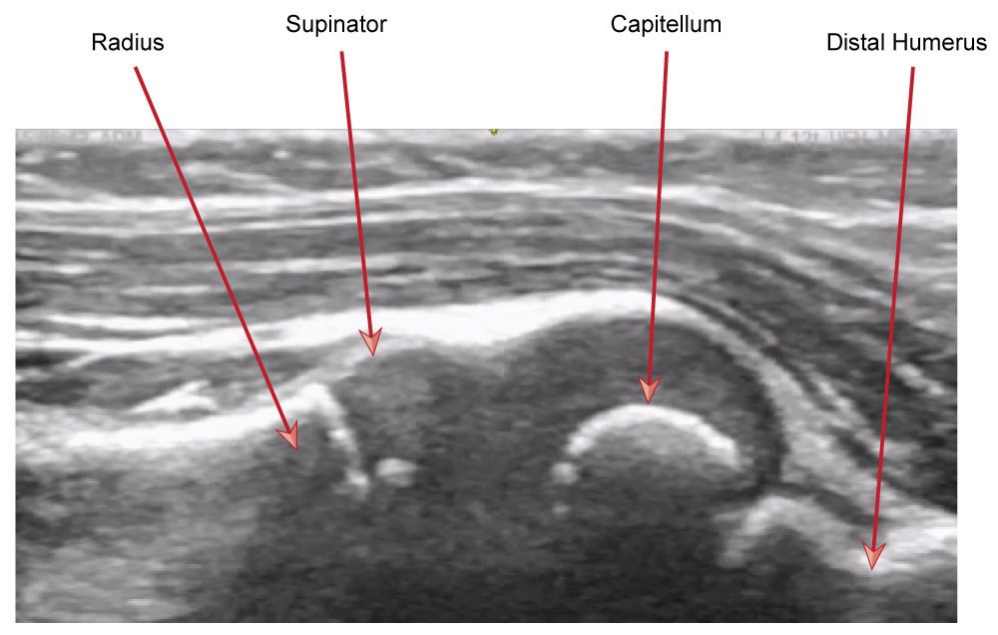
Sonographic anatomy – normal appearances. In this image the ultrasound probe is orientated caudally.
